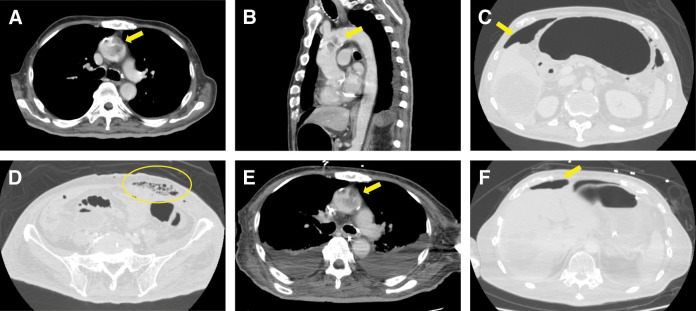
Contrast-enhanced CT findings of aortic arch mural thrombus without aortitis and concomitant deep port-site infection. On POD 10, axial and sagittal images of the aortic arch (A, B) show a coarse mural filling defect consistent with a large thrombus (arrows), without associated aortic wall thickening, abnormal mural enhancement, periaortic fat stranding, aneurysmal dilatation, or complex atherosclerotic plaque. Abdominal CT on POD 10 (C, D) demonstrates deep port-site infection, with gas tracking along the fascial plane and encapsulated fluid collections (contour line and arrows). Follow-up CT on POD 16 (E, F) shows a slightly smaller but persistent aortic arch thrombus and decreased intraperitoneal free gas (arrows), in line with partial resolution of the intra-abdominal septic focus.
